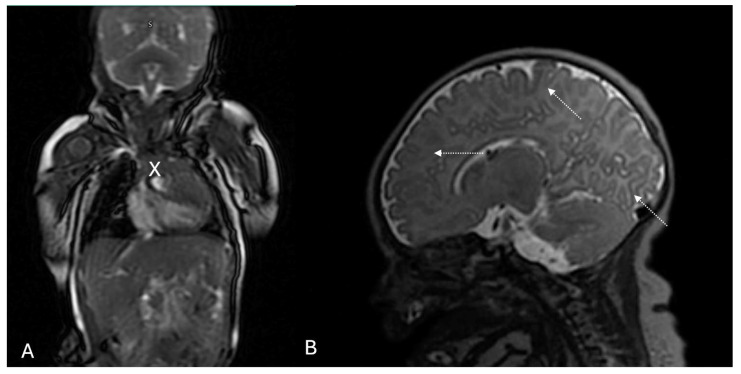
(A) Cardiac MRI in FLAIR sequence showing an oval-shaped, solid mass with well-defined borders, homogeneus appearance, and slightly hyperintense signals in T1 and T2, located in the anterior cardiac wall, measuring 33 × 33 × 15 mm (cross mark). (B) Additional T2 sagittal and T1 transverce views further confirm the presence of tubers (pointed arrows) and associated structural abnormalities in cortical-subcortical regions.
